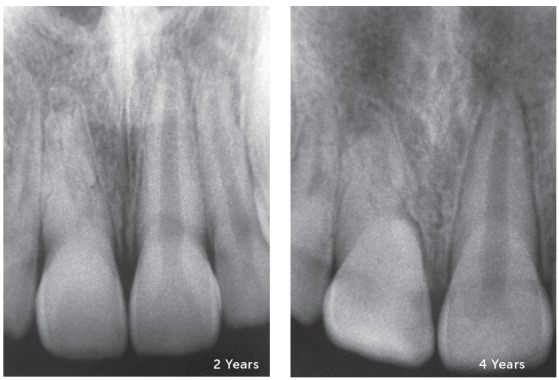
Replacement resorption in the maxillary central incisor, with preservation of vitality, as it can occur in dental trauma of lesser intensity of the concussion type. Radiographs were performed as control, after 2 and 4 years. The bone merges with the root structure, gradually replacing it.
